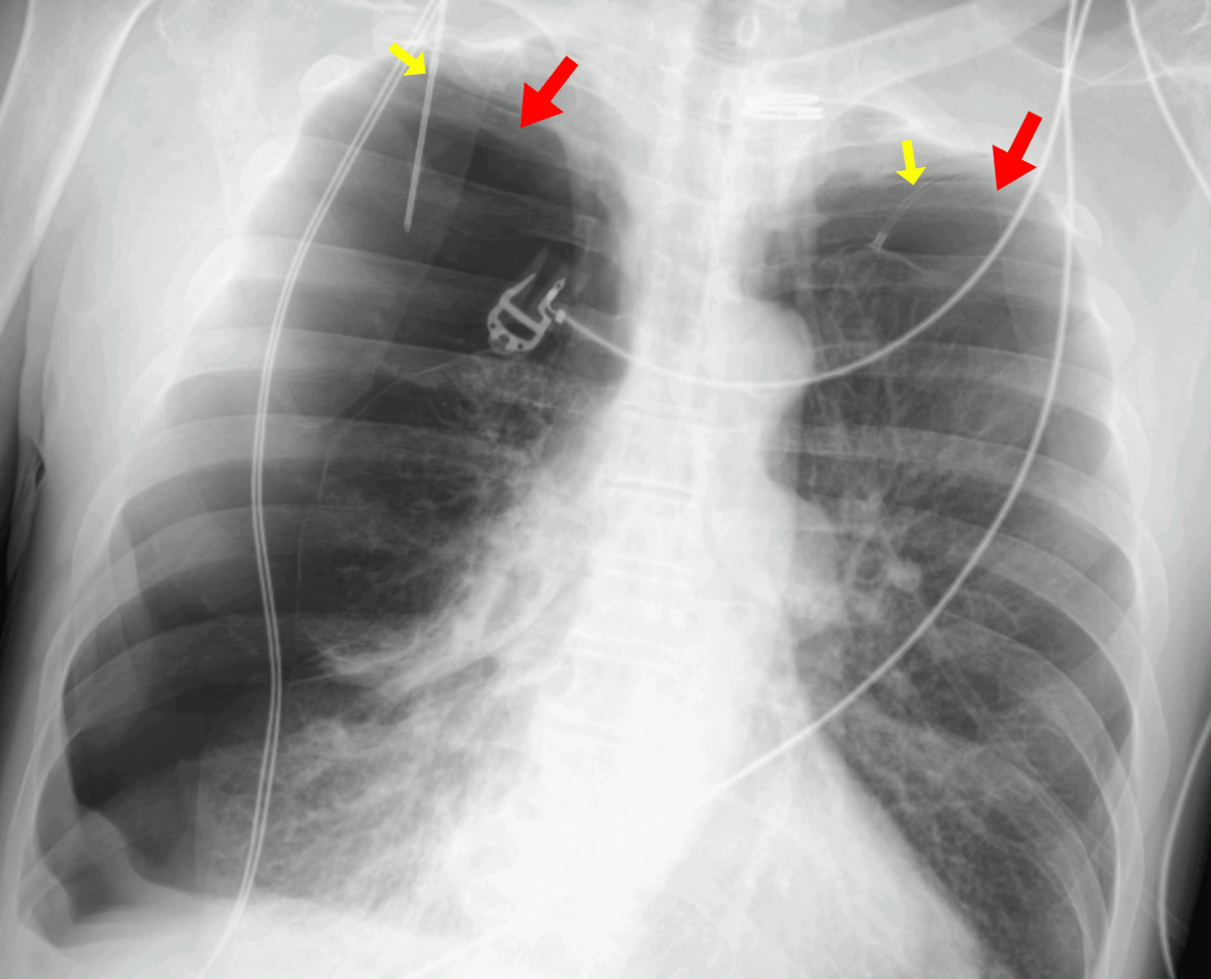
Chest radiograph demonstrating bilateral tension pneumothoraces following unilateral endobronchial valve placement Chest radiograph obtained after emergency bilateral needle decompression demonstrated bilateral pneumothoraces (red arrows), more pronounced on the right side, corresponding to the lung treated with endobronchial valve placement, with associated lung collapse. Needle decompression catheters are visible bilaterally (yellow arrows).
